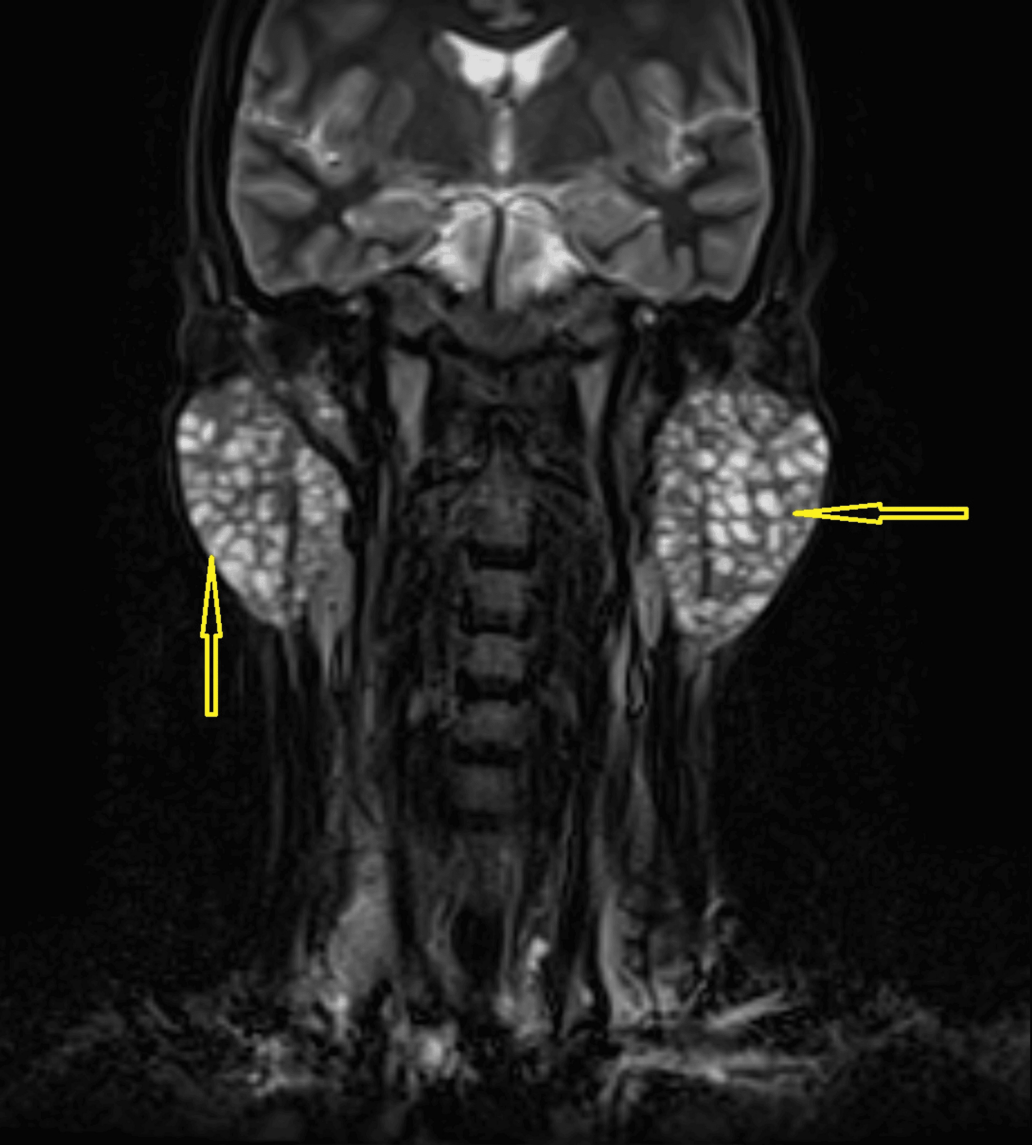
T2-weighted coronal image showing the hyperintense lesions in the bilateral enlarged parotid glands
